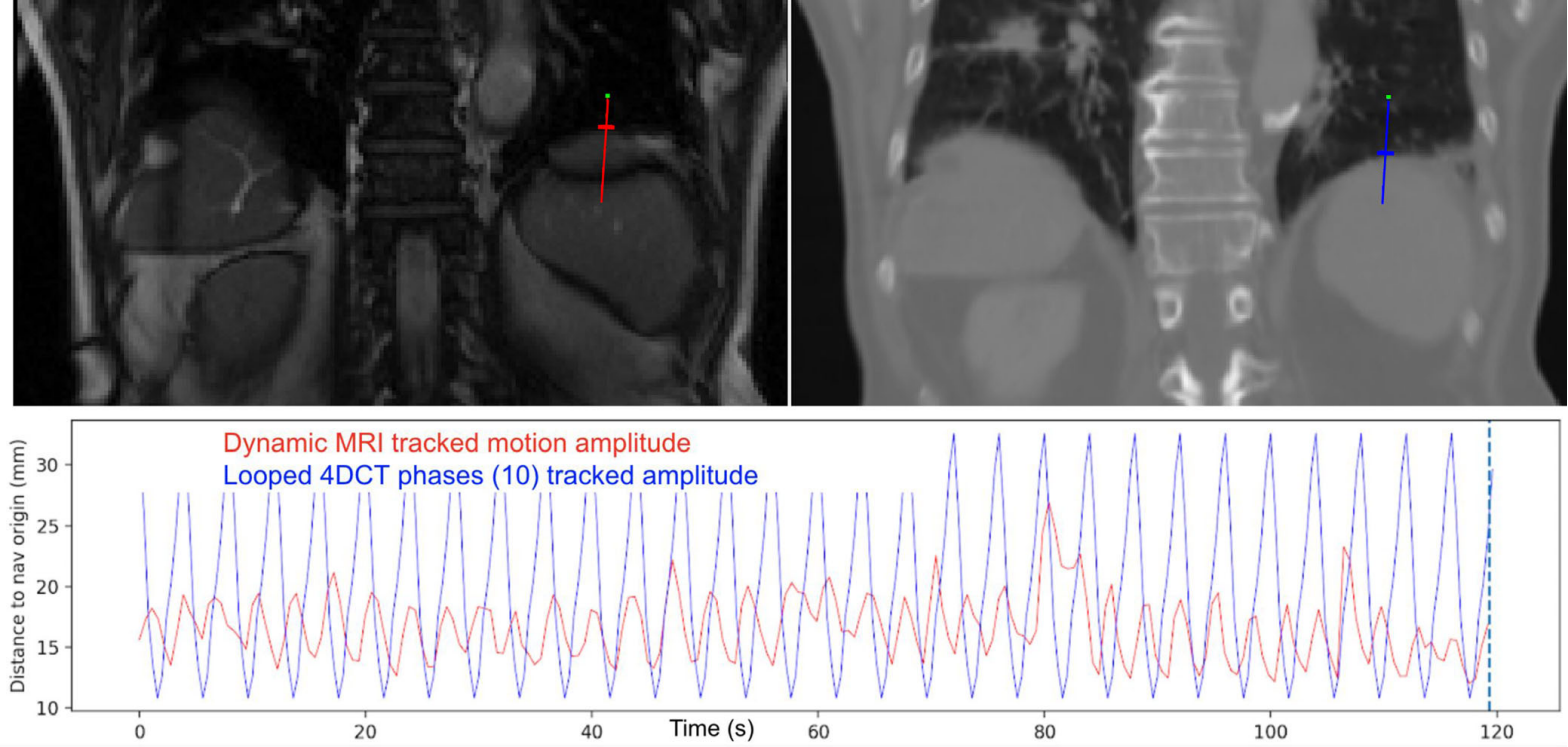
Example of motion amplitude tracked on both the 216 frames of the dynamic MRI (in red) and on the 10 looped frames of the 4DCTo (in blue). The tracked motion is given as the distance between the navigator end (green dot) and the tracked interface (small perpendicular line) in mm.
